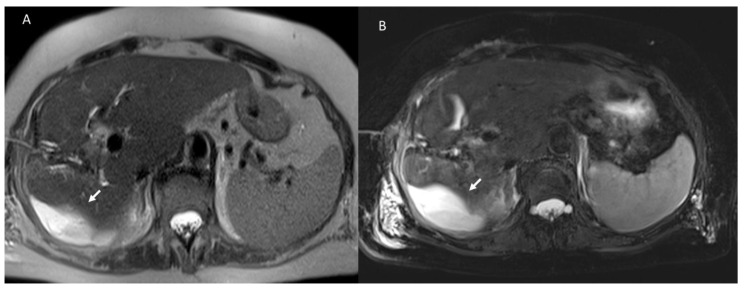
Postsurgical biloma assessed with MRI at 1 week post resection of VIII segment for liver metastasis. The biloma (arrow) appears hyperintense in T2 (A,B) sequences of MRI study.
